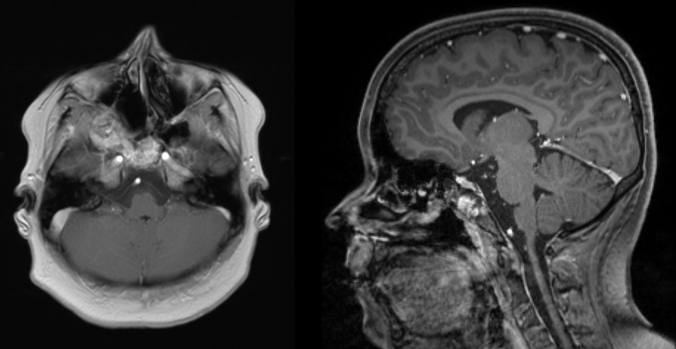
MRI of the head 18 months after primary surgery. Axial T1 weighted image (left) and sagittal T1 weighted image (right): tumor mass was significantly reduced after the first intervention. A minimal residual tumor affecting the clivus and the ethmoid bone can be observed but lesions do not cause any clinical symptoms.
